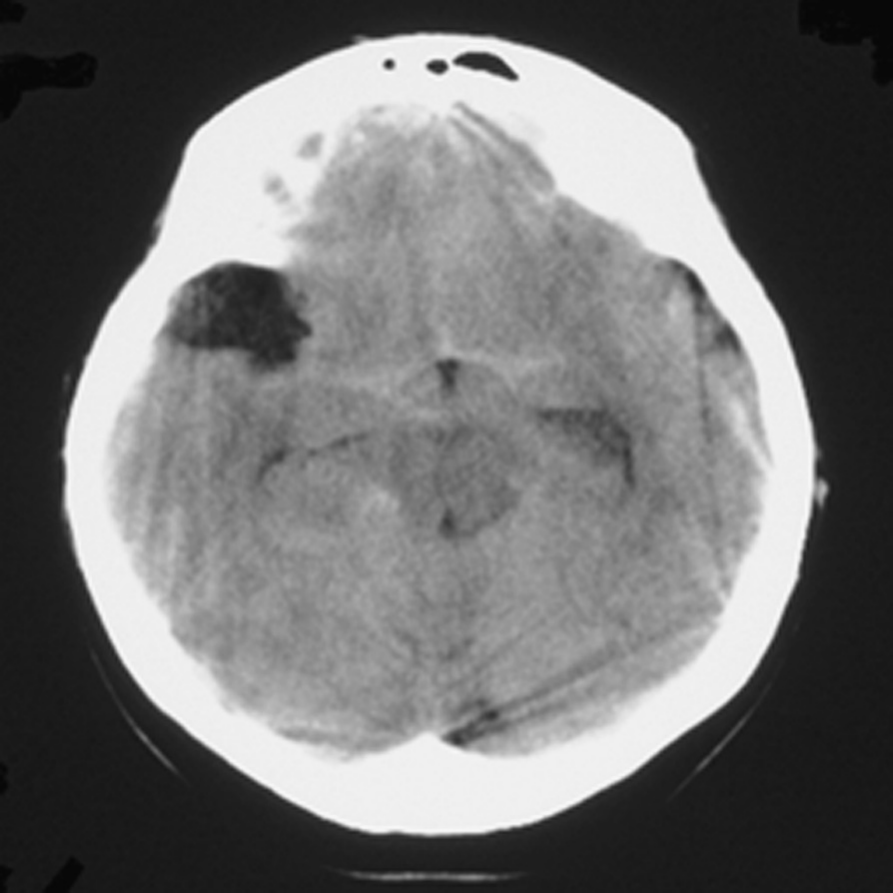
Computed tomography scan of the brain on arrival at the hospital, indicating diffuse subarachnoid hemorrhage and an arachnoid cyst at the right middle fossa.
